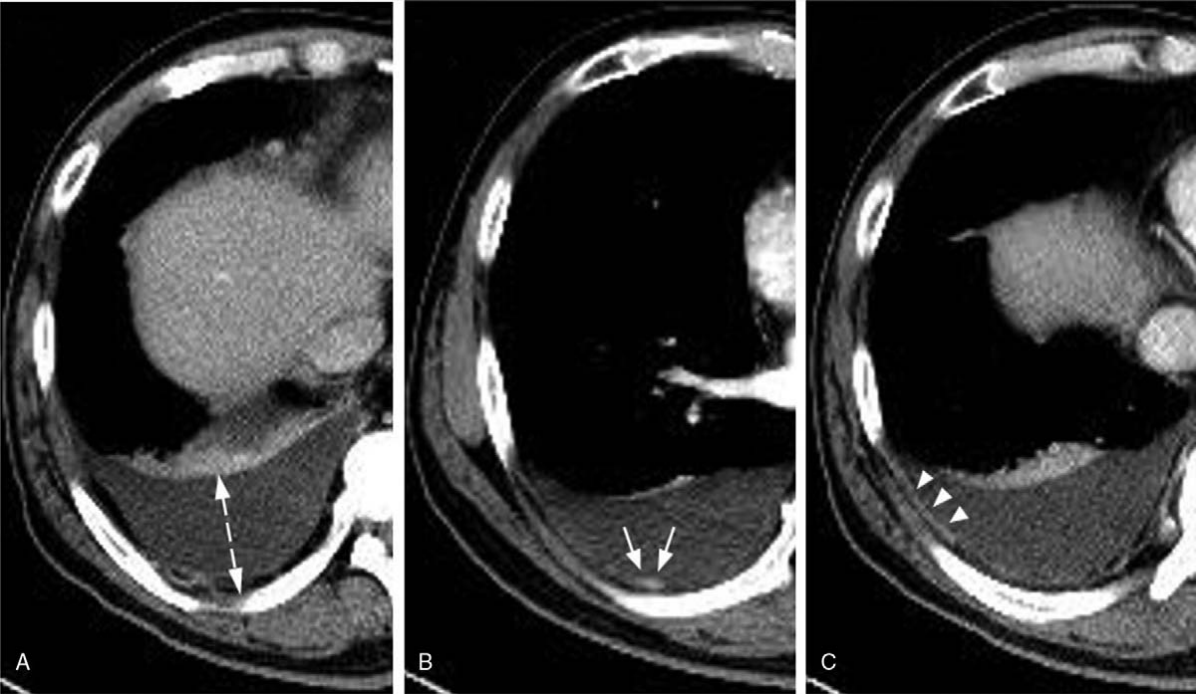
Chest computed tomography (CT) of MPE. (A) The depth of MPE was measured perpendicular to the parietal pleura at the site of greatest depth on axial CT images (dashed arrow). (B) CT image of MPE with pleural nodularity (arrow). (C) Chest CT showing pleural thickening with pleural enhancement (arrowhead). MPE = malignant pleural effusion.
